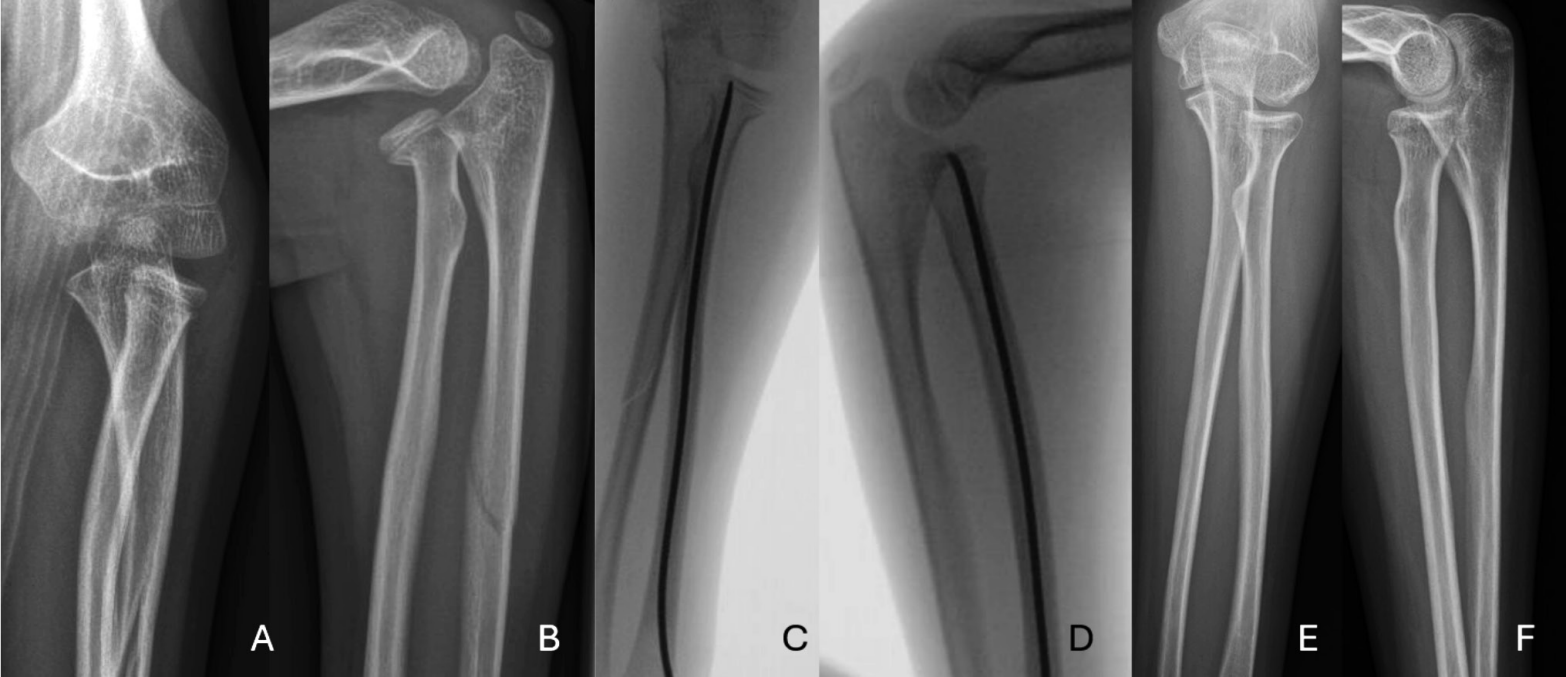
A 10-year-old boy, with left hand injury. In the radiographs a proximal fracture of the radius and anterior luxation of the radiohumeral joint and ulnar diaphyseal fracture were registered (A, B). This was classified as Bado type IV Monteggia fracture. Injury was treated with flexible intramedullary nail (C, D). 5 years later after removal of the nail, the injury had recovered fully radiologically and clinically (E, F).
